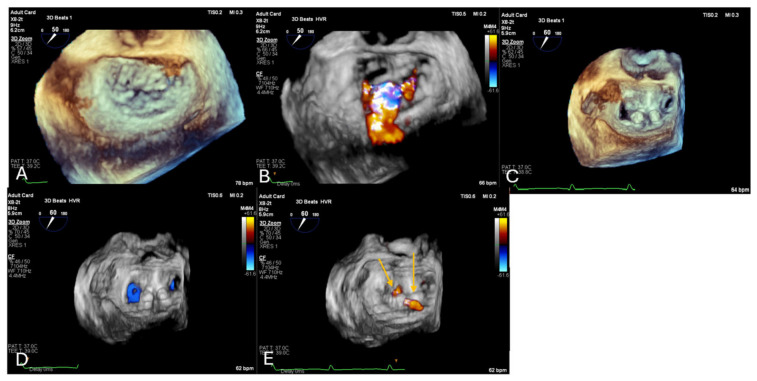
Severe secondary mitral regurgitation. 3D zoom from the left atrium perspective (A) with Color Doppler (B). After 2 PASCAL device implantations, 2 new mitral valve orifices were demonstrated
